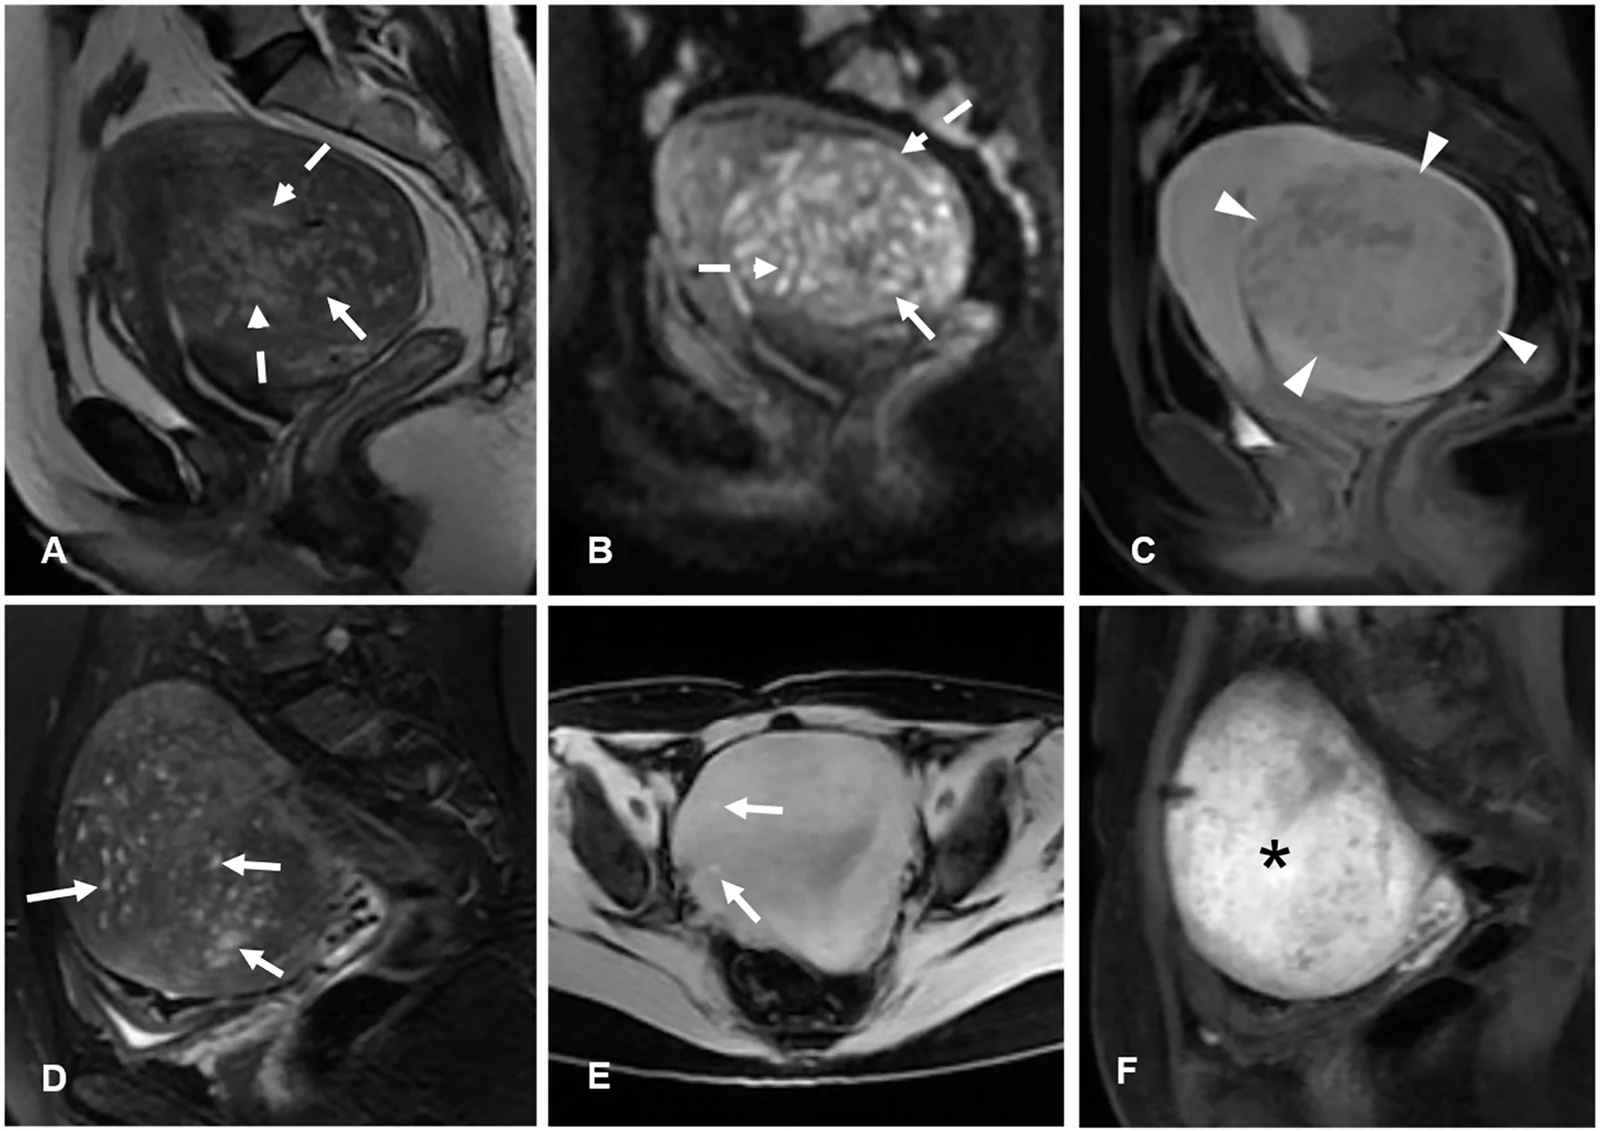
Magnetic resonance images of LG-ESS (A–C) and adenomyosis (D–F) in a 50-year-old woman. Sagittal T2W (A) and DW (B) images demonstrate a mass in the posterior uterine wall with ill-defined margins. Within the mass, “worm-like” (dotted arrows) and nodular (arrows) areas of high signal intensity are visible on both T2WI and DWI. Sagittal contrast-enhanced T1-WI (C) shows relatively clear delineation of the tumor margins and enhancement slightly lower than that of the surrounding myometrium. Sagittal T2W image (D) shows uterine enlargement with a thickened uterine wall containing multiple nodular T2WI hyperintense foci. Axial T1-WI (E) reveals punctate high-signal foci within the lesions. Sagittal contrast-enhanced T1-WI (F) shows lesion enhancement similar to that of the myometrium, without clear boundaries
